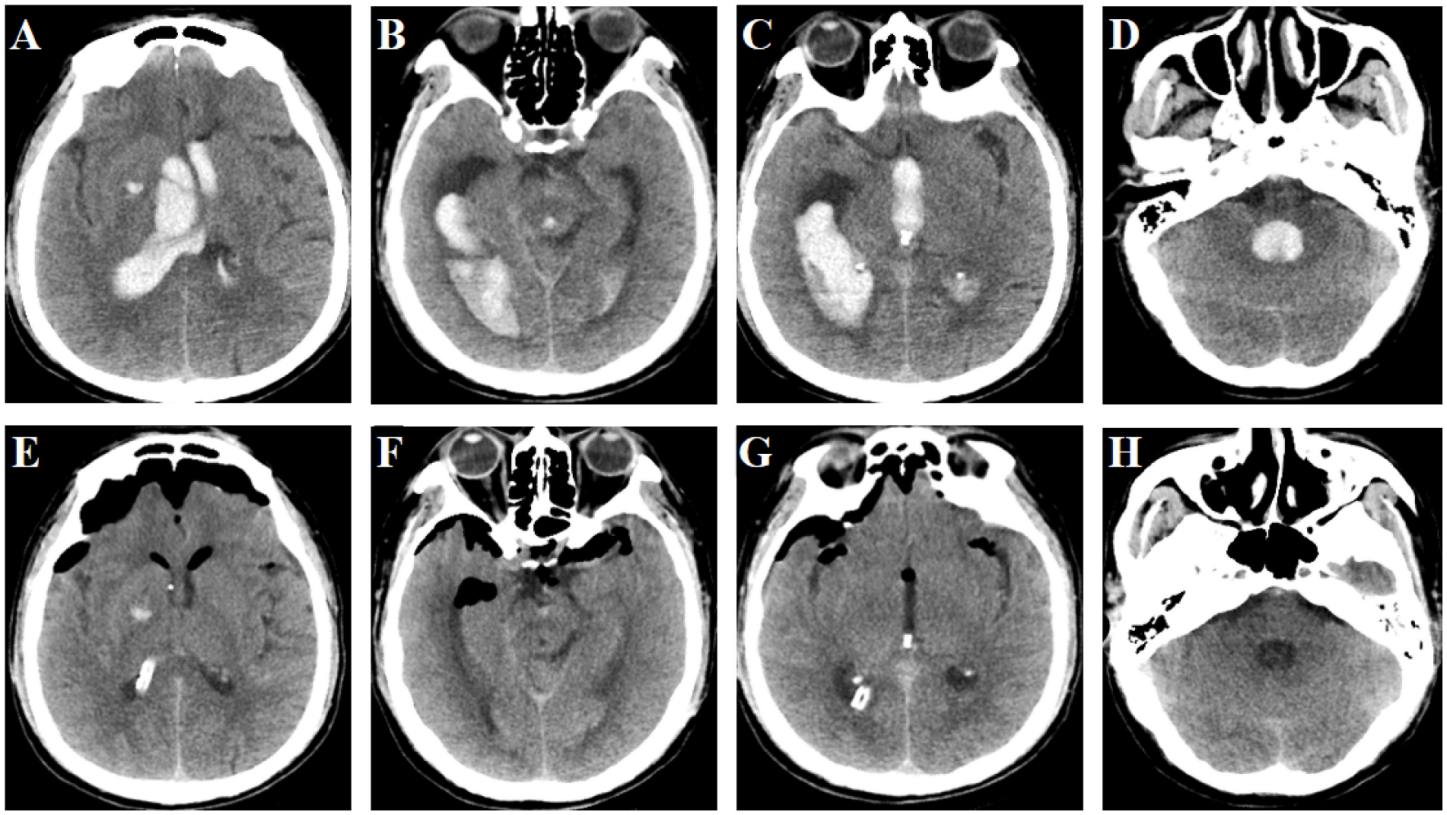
Pre- and post-operation CT scans of an irregular intraventricular hemorrhage that was evacuated by FATSE combined with a “Two-in-One” neuroendoscopic approach. (A) Preoperative CT scan showing the hematoma was located in the body of the lateral ventricles. (B) Preoperative CT scan showing the hematoma was extended to temporal horn of lateral ventricles. (C) The hematoma extended to the third ventricle. (D) The hematoma extended to the fourth ventricle. (E) CT scan showing the hematoma was completely evacuated in the body of the lateral ventricles. (F) CT scan showing evacuation of hematoma in temporal horn of lateral ventricles. (G) CT scan showing the hematoma was completely evacuated in the third ventricle (H) CT scan showing the hematoma was completely evacuated in the fourth ventricle.
